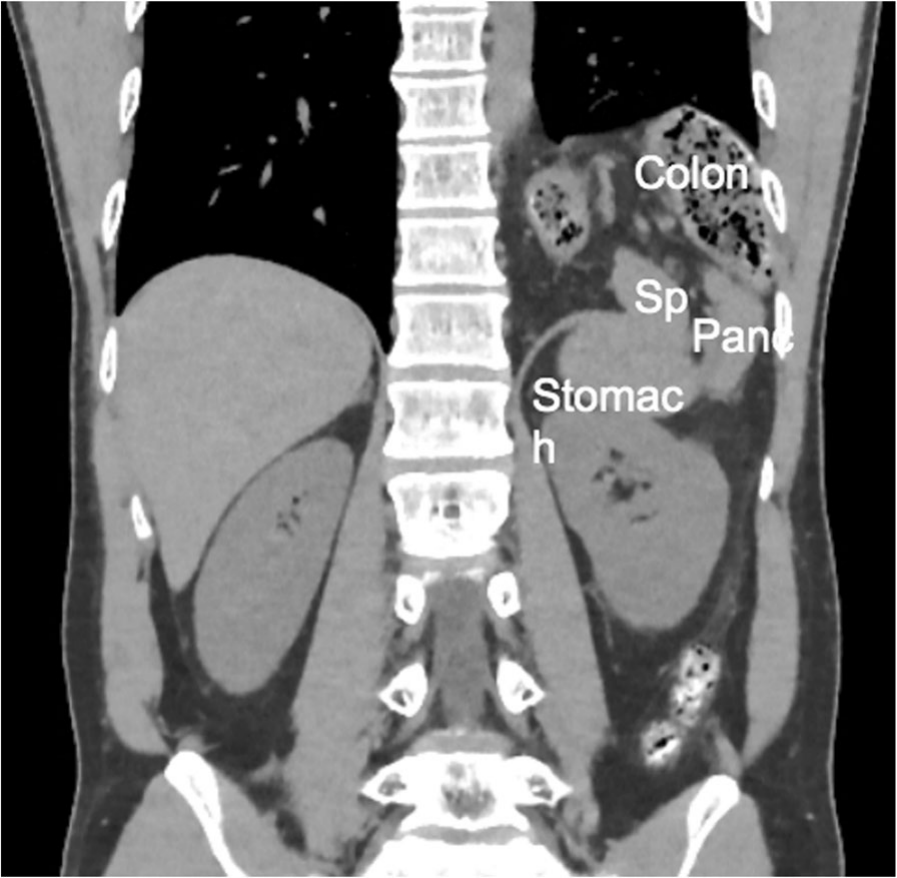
CT imaging 10 years before surgery showed modestly prolapsed viscera (colon, spleen, and pancreas)
